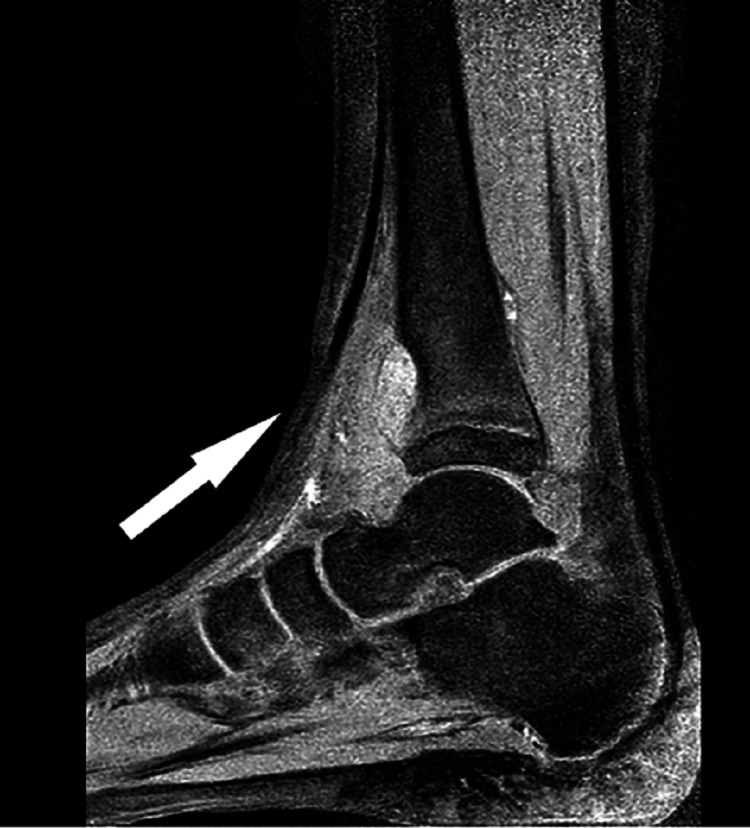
Sagittal view of right lower limb MRI demonstrating an abscess in the distal tibia breaching the cortex and extending into the surrounding soft tissue
